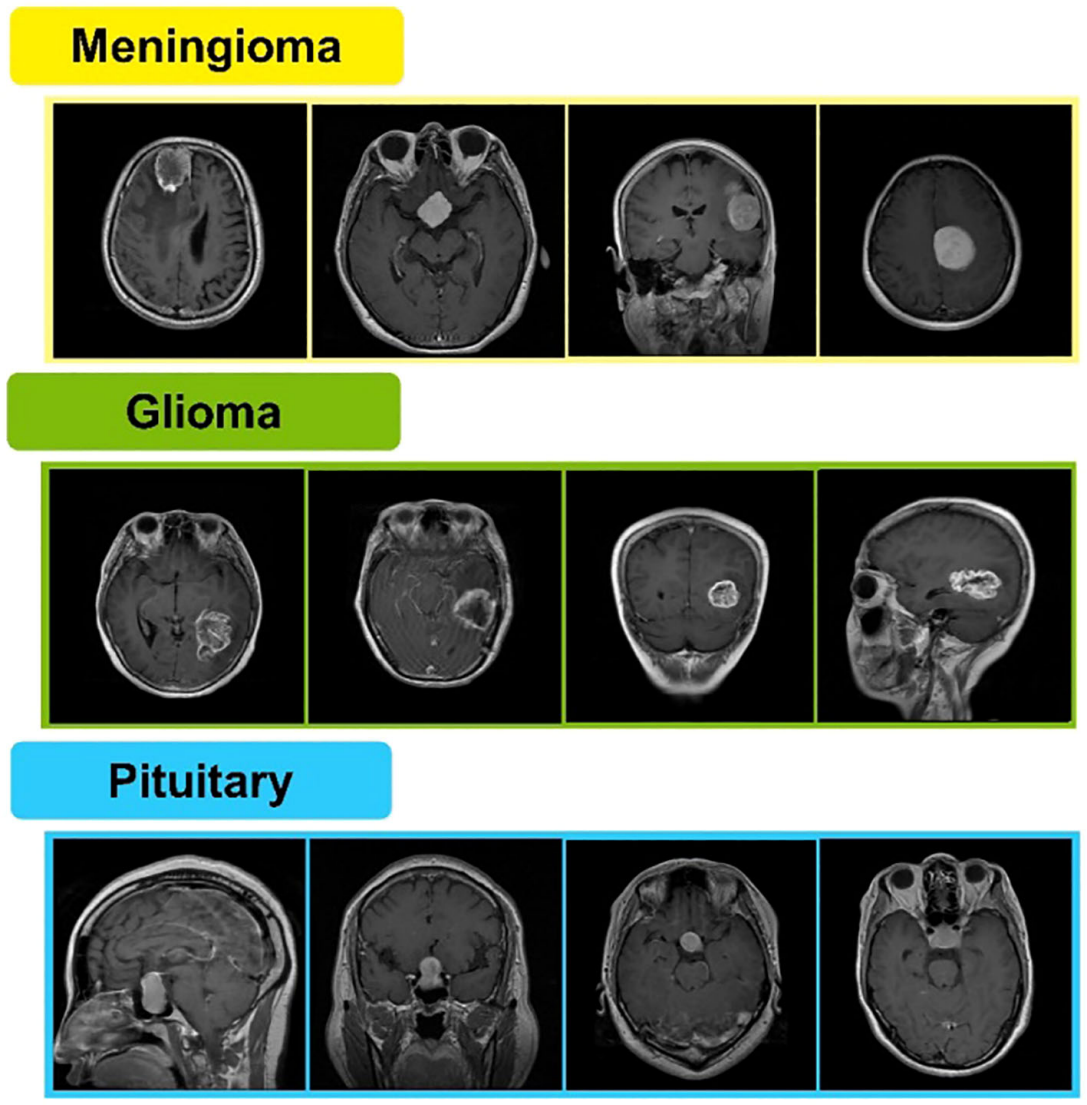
Sample MRI images of brain Meningioma, glioma, and pituitary tumors.
